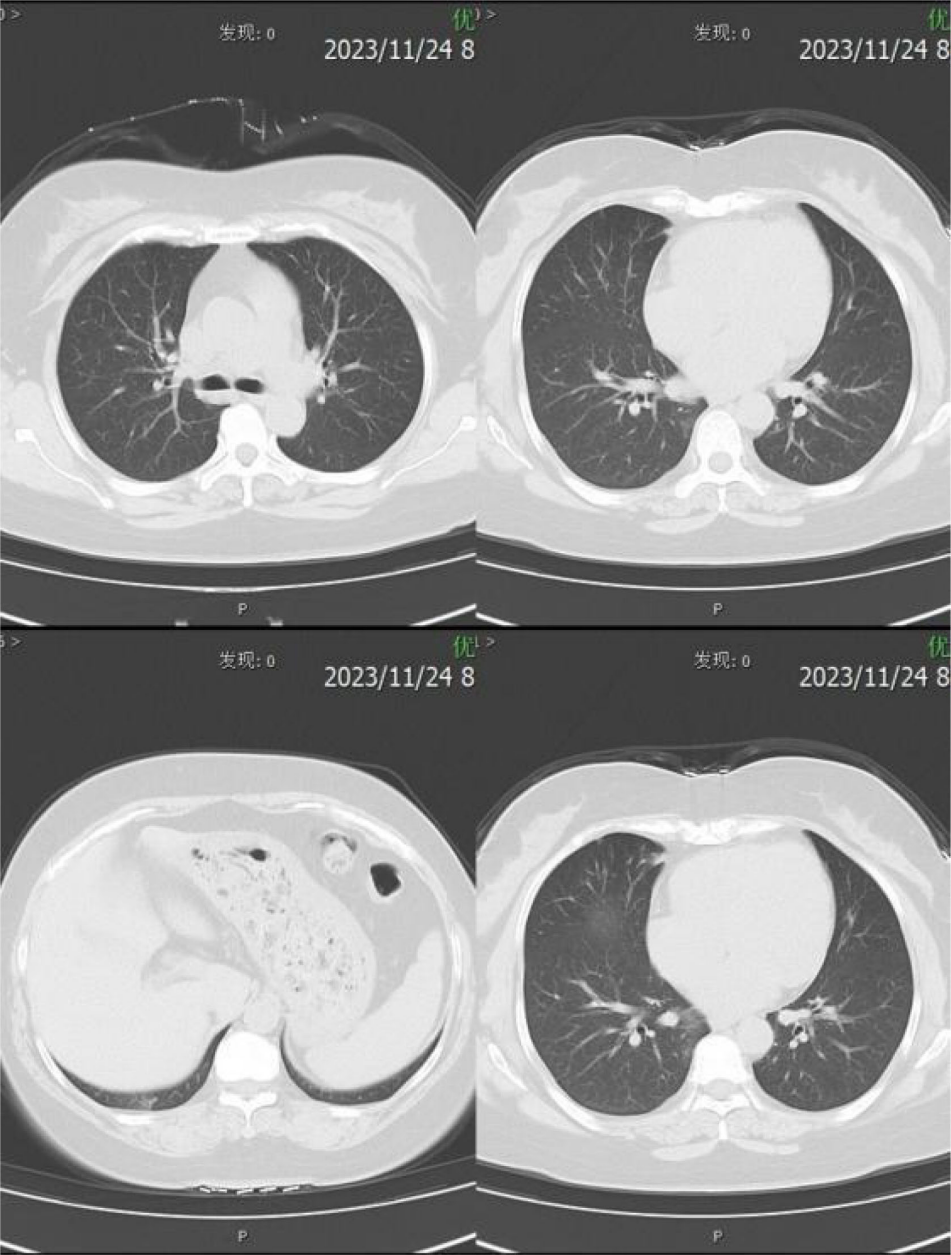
CT imaging of the chest.
